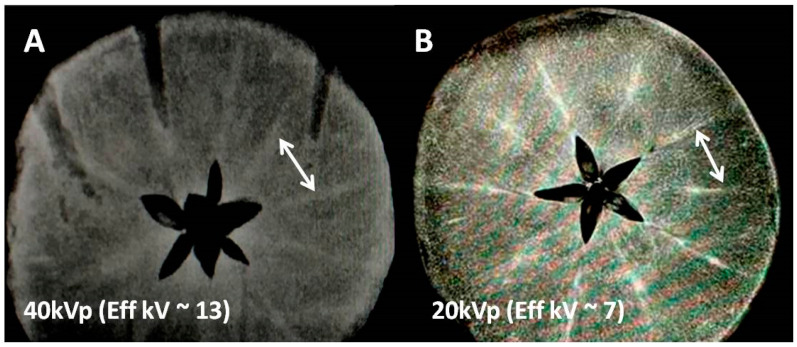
X-ray images of the mineral-rich apple at two different X-ray energies showing mineral conspicuity as a function of mean X-ray energy. (A) Non-contrast X-ray image at 40 kVp/10 mAs (with mean energy approx. 13 keV) using a clinical scanner; two typical high attenuation tracks marked by a white arrow indicate either K or Fe concentrated regions (Table 1). (B) Another apple slice showing the same mineral tracks at higher mineral conspicuity presumably due to lower kVp (soft) mammographic X-ray beam (20 kVp/10 mAs with mean energy approx 7 keV, close to k-edges of transition metal ions but far from k-edge of K ions, Table 1).
